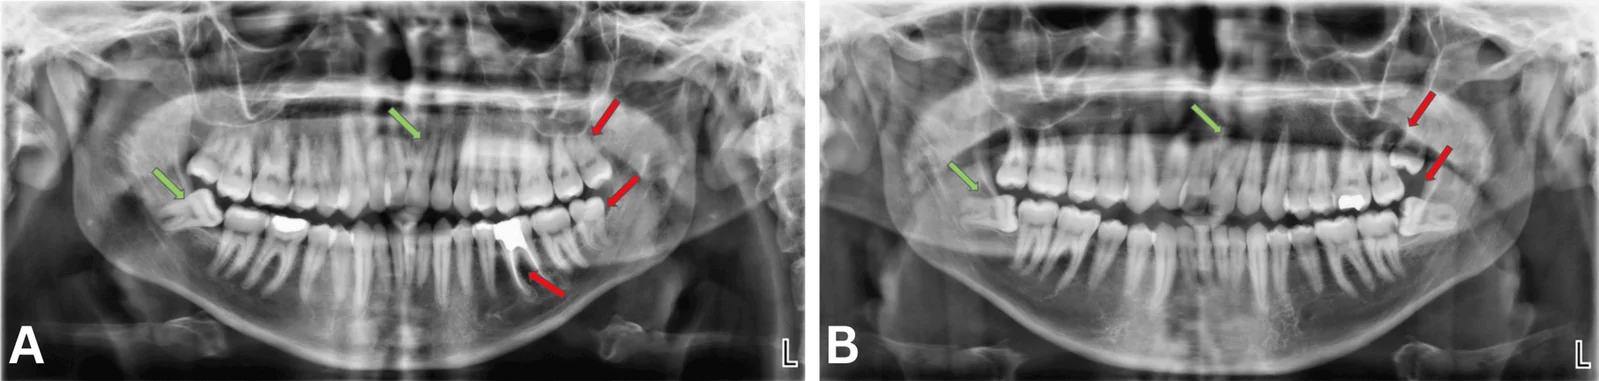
Panoramic (orthopantomogram) radiographs of (A) T1 and (B) T2 Green arrows indicate shared radiographic findings, whereas red arrows indicate differences between the twins. T1: Twin 1, T2: Twin 2
